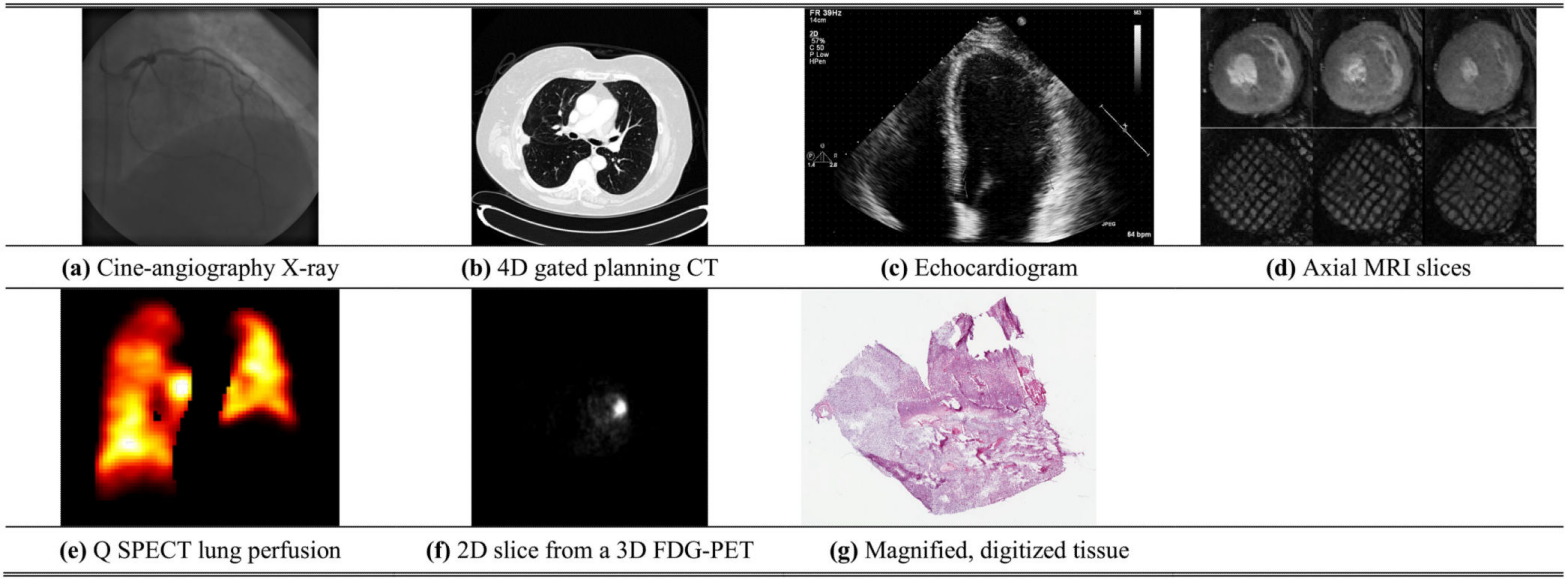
Typical medical imaging examples. (a) Cine angiography X-ray image after injection of iodinated contrast; (b) An axial slice of a 4D, gated planning CT image taken before radiation therapy for lung cancer; (c) Echocardiogram – 4 chamber view showing the 4 ventricular chambers (ventricular apex located at the top); (d) First row – axial MRI slices in diastole (left), mid-systole (middle), and peak systolic (right). Note the excellent contrast between blood pool and left ventricular myocardium. Second row –tissues tagged MRI slices at the same slice location and time point during the cardiac cycle. The modality creates noninvasive magnetic markers within the moving tissue [40]; (e) A typical Q SPECT image displaying lung perfusion in a lung-cancer patient; (f) A 2D slice from a 3D FDG-PET scan that shows a region of high glucose activity corresponding to a thoracic malignancy; (g) A magnified, digitized image of brain tissue to look for signs of Glioblastoma (taken from TCGA Glioblastoma Multiforme collection (https://cancergenome.nih.gov/).
